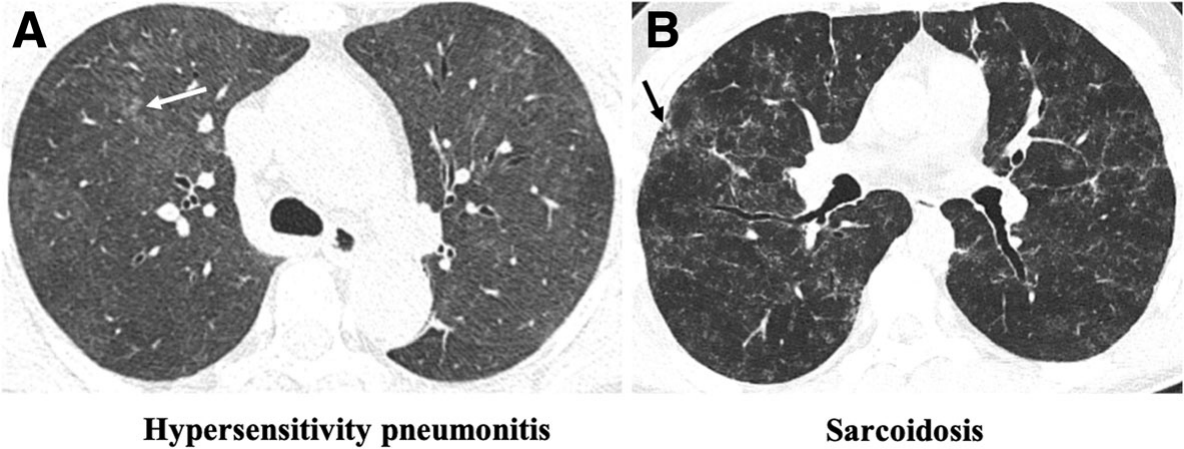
Differential diagnosis of RB-ILD. a Axial CT image depicts subacute hypersensitivity pneumonitis (HP) in a 71-year-old woman with a long history of exposition to moldy hay (farmer’s lung). Imaging findings of HP are similar to RB-ILD, which also appears as centrilobular nodules predominantly in the upper lobes (arrow) and patchy areas of ground-glass attenuation. A clinical history is crucial to make the differential diagnosis. b Sarcoidosis in a 37-year-old man. Axial CT image shows diffuse areas of nodularity with peribronchial distribution and also in relation to the subpleural region (arrow). There are also some surrounding ground-glass opacities
